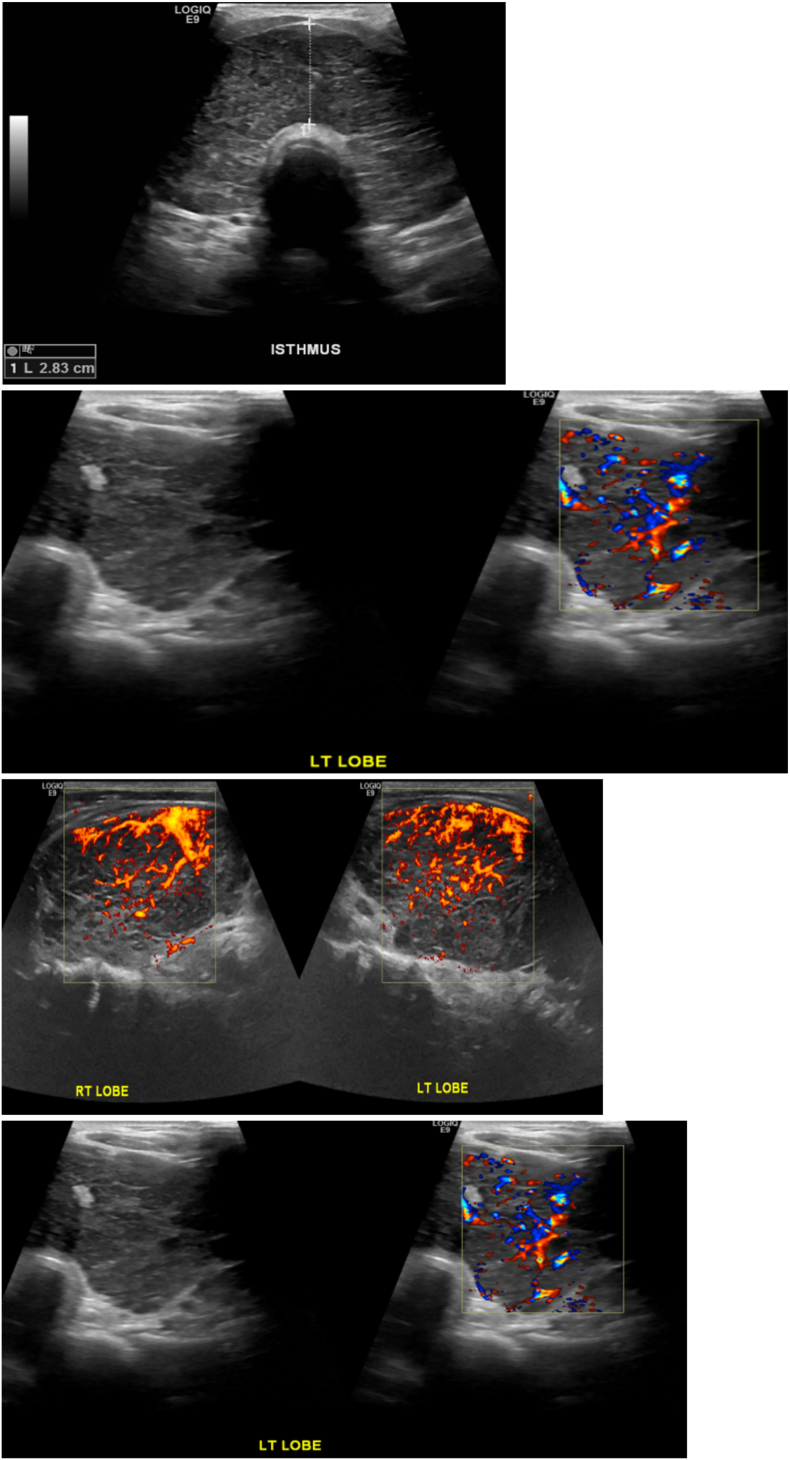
Thyroid ultrasound: thyroid parenchyma shows diffuse heterogenous echotexture pattern and increased vascularity.
